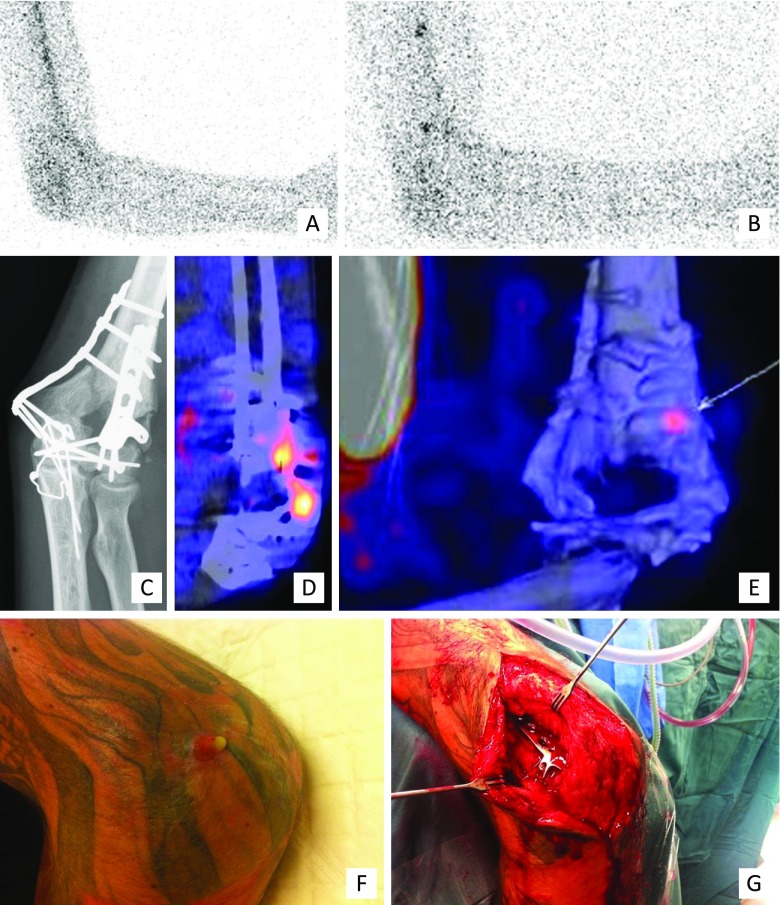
Clinical example of WBC scintigraphy + SPECT/CT. A 37-year-old man with a grade 3A complicated distal humeral fracture of the left elbow, initially treated with an external fixator and subsequently by plate osteosynthesis of the distal humerus. c X-ray: situation after recent fixation of the fracture with plate osteosynthesis, no signs of loosening or infection. After 4 months, he presented with a fistula and a clinical suspicion of osteomyelitis of the distal humerus. a–b, d–e: WBC scintigraphy (a image at 4 hours, b image at 24 hours, d–e fusion SPECT/CT images) after the injection of 220 MBq 99 m-Tc-labeled leucocytes demonstrated an infection around the implant at the lateral side of the elbow/distal screw. The low uptake points to only a low-grade appearance and the location to soft tissue involvement; this was confirmed at operation (f clinical pre-operative picture, g perioperative clinical picture)
